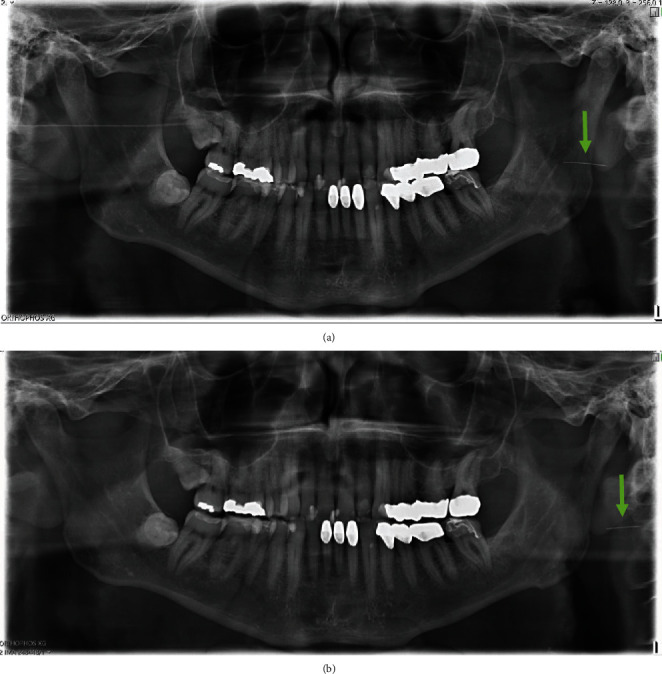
Orthopantomographic images on the day of initial presentation (a) and one day afterwards (b). The dislodged fractured hypodermic needle is located at the left ascending mandibular ramus and moved by about 1.5 cm after one day.
